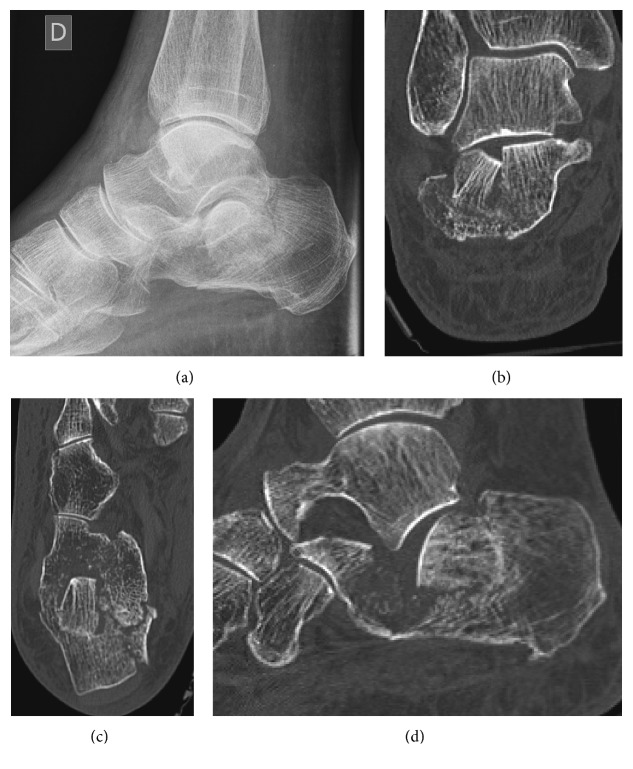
Preoperative radiographs and CT scan of the right foot, confirmed a bony destructive Sanders type IIIBC fracture with a Boehler's angle of less than 5 degrees.
